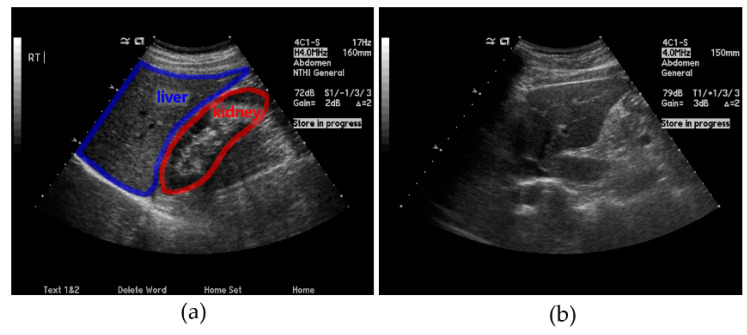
Examples of ultrasound (US) images: (a) parasagittal and (b) non-parasagittal.
